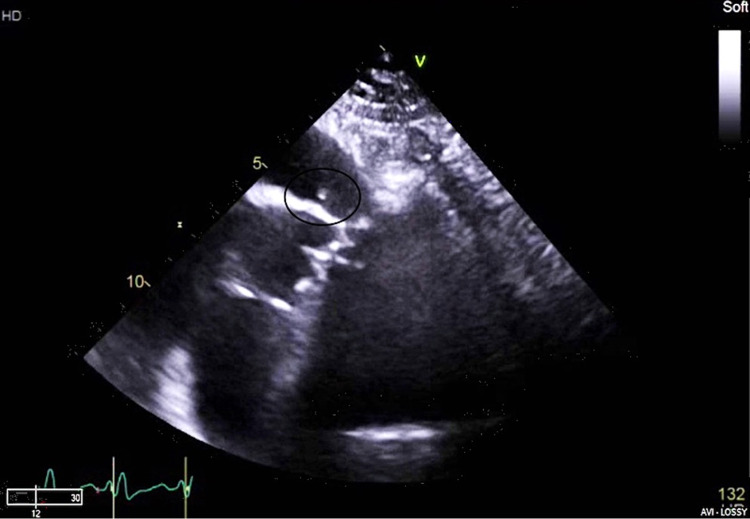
Trans-thoracic echocardiogram modified basal short-axis view showing a mobile 5 mm vegetation on the sub-pulmonic ridge.
